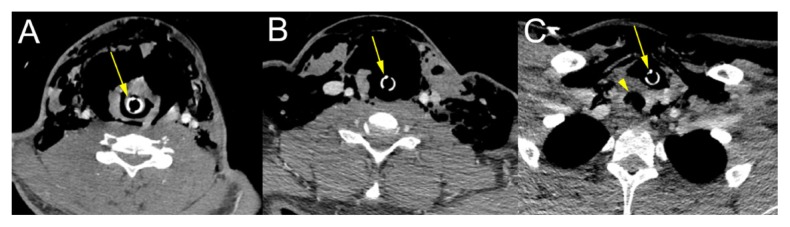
Transverse images of a CT of the neck show path of the ET tube (arrow): A) CT scan showing ET tube passing through glottis; B) ET tube moving inferior, anterior airway noted to have possible disruption; and C) ET tube is noted to be outside of the trachea (arrowhead).
